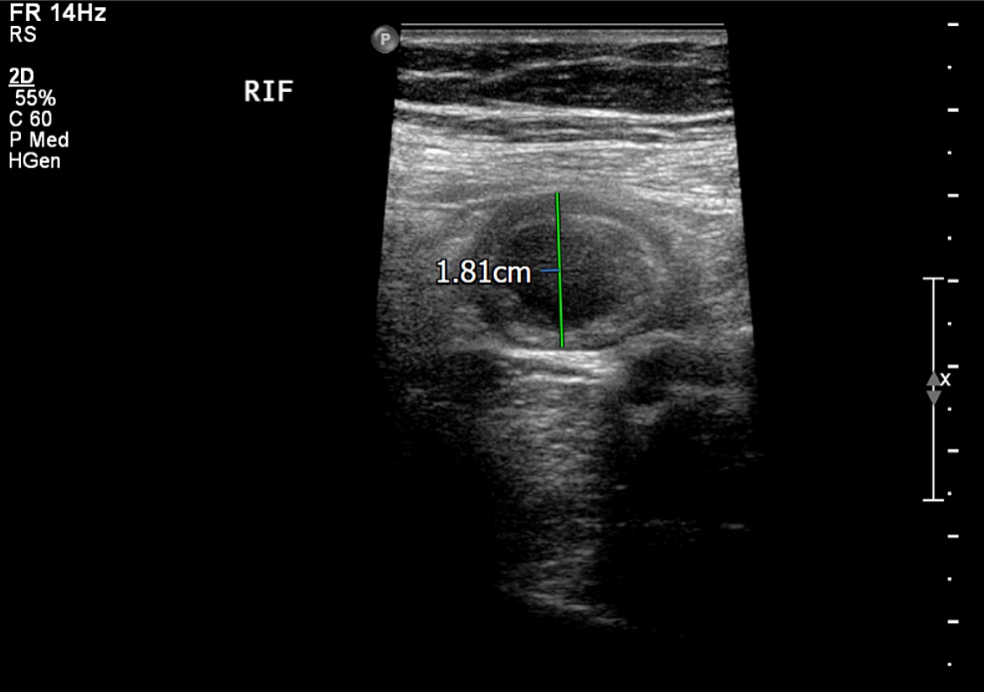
Abdominal ultrasound scan showing a thick-walled bowel loop (measuring up to 8 mm) suggesting inflammation.
